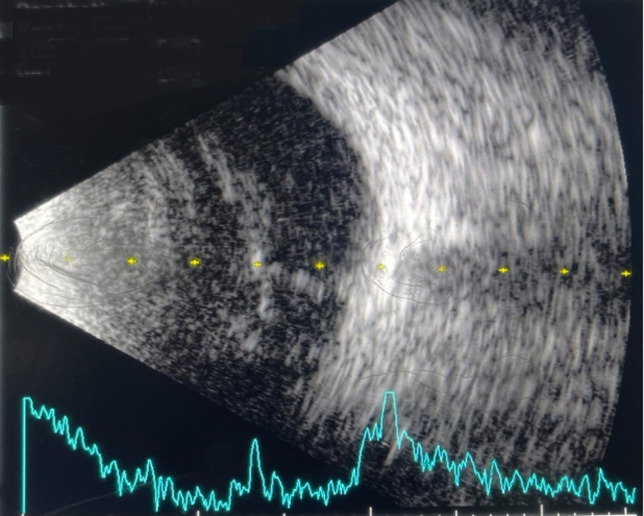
ultrasonography or the right eye revealed membrane-shaped echogenic lesion with minimal mobility and high echo spike with numerous particles beneath the membrane
